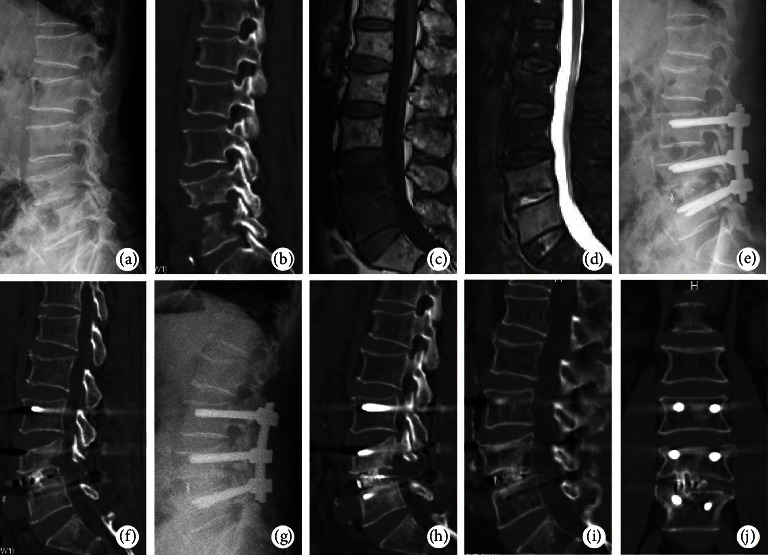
A 68-year-old male with lumbar pyogenic spondylodiscitis. (a) Preoperative lumbar spine X-ray. (b) Preoperative lumbar CT image. (c) T1-weighted image showed hypointensity of L4–L5 vertebral body. (d) T2-weighted fat-suppression sequence showed relative homogeneous enhancement of vertebral bodies and remarkable hyperintensity of the disc. (e, f) Postoperative lumbar radiological images. (g, h) Radiological images at 3 months postoperatively. (i, j) The CT scan showed solid interbody fusion at 1 year after surgery.
